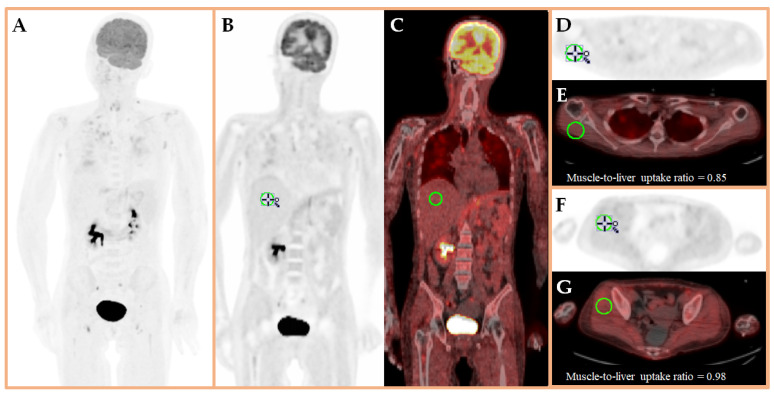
The paraneoplastic and pembrolizumab-induced myositis were treated with intravenous immunoglobulin (2 g/kg) and prednisolone at an equivalent daily dose of 50 mg [7,8,9]. The prednisolone dose was gradually tapered to 5 mg daily over a two-month period. The patient subsequently underwent a modified radical mastectomy, with a final pathological stage of ypT2N1a. Follow-up ^18^F-FDG PET performed 5 months after the initial scan revealed reduced muscular ^18^F-FDG uptake (A–C), with scapular (D,E) and gluteal (F,G) muscle-to-liver SUV_mean_ ratios of 0.85 and 0.98, respectively. The creatine kinase level normalized, and her symptoms gradually subsided. She could walk using a walker and required minimal assistance with self-care. Myositis is a rare comorbidity among patients with TNBC, whether as an immune-related adverse event of pembrolizumab or as a paraneoplastic manifestation. In this case, the onset occurred immediately after the first cycle of pembrolizumab, suggesting that the pembrolizumab may trigger a latent paraneoplastic phenomenon. While serum creatine kinase levels are routinely used as a biomarker of muscle injury, ^18^F-FDG PET enables direct visualization of inflammatory activities, allows assessment of myositis severity, and assists in distinguishing myositis from cancer metastasis. This case demonstrates the value of ^18^F-FDG PET as a molecular imaging modality to evaluate patients with cancer with suspected paraneoplastic or immune checkpoint inhibitor-associated myositis.
